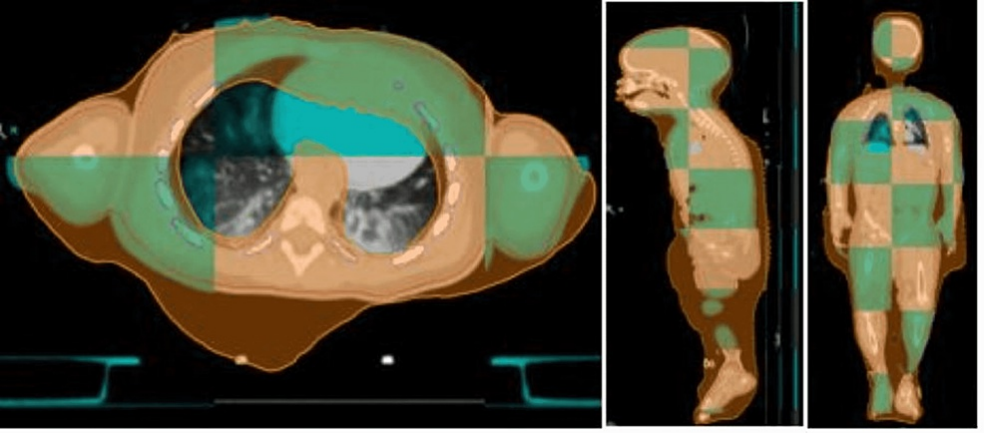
Megavoltage CT (MVCT) - planning CT image registration in axial (left), sagittal (middle), and coronal (right) view. The MVCT image shows the body is within the 95% isodose colorwash displayed in orange.
